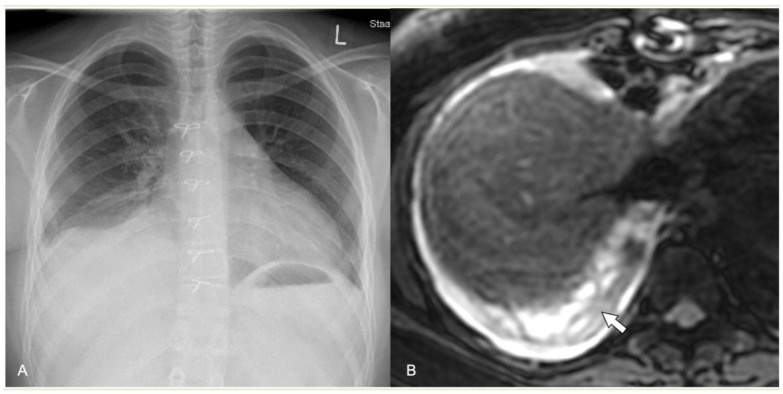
Complicated pneumonia following heart surgery. (A) Chest radiograph demonstrates the blunting of right pleurodiaphragmatic angle and a possible subpulmonary pleural effusion. (B) Axial MRI, T2-weighted sequence. Note the clear depiction of a pleural effusion with septations (arrow).
